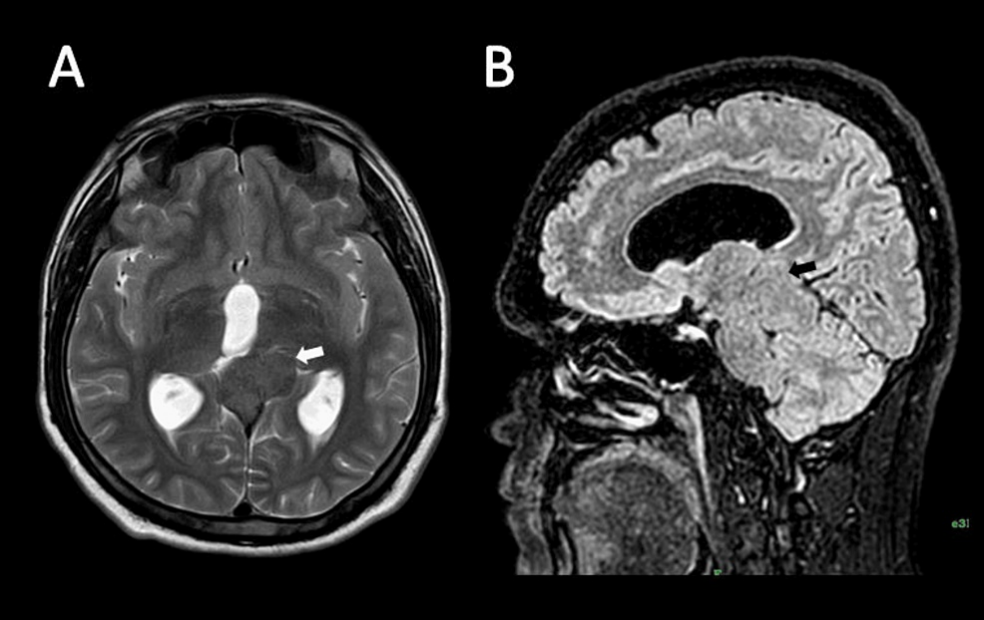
Case 1, a 40-year-old woman. T2-weighted and FLAIR sequences. (A) Axial T2-weighted image shows a well-defined, lobulated, extra-axial, pineal region mass isointense to mildly hypointense relative to gray matter. This lesion causes a mass effect on the dorsomedial aspect of the left thalamus (white arrow). (B) The sagittal FLAIR image shows this lesion (black arrow) is mildly hypointense to gray matter. FLAIR: fluid-attenuated inversion recovery
